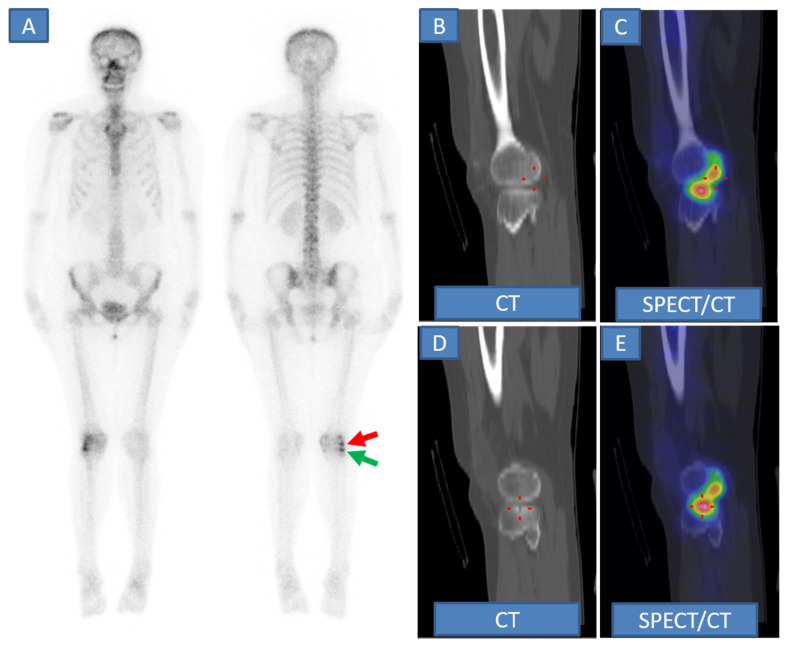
Bone SPECT/CT showed bony destruction, cleft formation, and focal radiotracer uptake in the distal femur ((A), red arrow; (B,C), crosshairs) and proximal tibia ((A), green arrow; (D,E), crosshairs), SPECT/CT with rainbow color scale. Combined imaging and arthroscopic findings supported femoral osteochondritis dissecans (OCDi) and a tibial osteochondral defect (OCDe). Cartilage lesions may occur alone or with subchondral bone involvement, the latter termed OCDe [1]. Osteochondral lesions (OCL) represent focal abnormalities of articular cartilage, the subchondral plate, and marrow, and arise from trauma, subchondral insufficiency fractures, avascular necrosis, osteoarthritis-related collapse, or OCDi [1,2,3]. OCDe commonly results from acute injury or repetitive microtrauma leading to microtrabecular fractures and impaired remodeling [3]. Early lesions typically show marrow edema, subtle cortical disruption, or hypointensity beneath the subchondral plate on MRI [2,4], whereas advanced lesions may develop contour deformity, instability, or loose bodies, increasing the risk of early osteoarthritis [3]. Although both OCDe and OCDi involve the osteochondral unit, they remain distinct. OCDe reflect localized structural loss of cartilage and subchondral bone from acute or chronic mechanical injury without a primary vascular mechanism [4]. In contrast, OCDi results from subchondral ischemia causing necrosis and potential osteochondral fragment (loose body) separation [1,5]. OCDi occurs more often in adolescents due to the vulnerability of the immature epiphysis [6]. Clinically, the acronym “OCD” is often misused to describe both conditions; however, it should strictly denote osteochondritis dissecans [2]. In this report, we use the term “OCDi” to clearly distinguish osteochondritis dissecans from OCDe, a specific subtype within the broader category of OCL. Before CT and MRI were widely used, Tc-99m phosphate scintigraphy proved valuable for detecting juvenile OCDi, owing to its sensitivity to lesion activity [7]. Bone scintigraphy remains a useful screening tool for persistent bone pain, though planar imaging has limited localization [8]. SPECT/CT or quantitative scanning improves anatomical precision and metabolic characterization, with reported value in identifying ankle and knee OCDi lesions [5,8]. Arthroscopy is reserved for persistent symptoms and to evaluate structural lesion stability across established grading systems [9,10]. OCDi is a specific osteochondral pathology caused by vascular compromise, most commonly in the knee (especially the medial femoral condyle); adult lateral femoral condyle involvement, as in this case, is uncommon but clinically important. Bone SPECT/CT is valuable for both OCDe and OCDi, demonstrating focal uptake, clefts, and subchondral destruction while providing metabolic detail. Early and accurate diagnosis is essential for appropriate management in adults with persistent knee pain unresponsive to conventional therapy.
